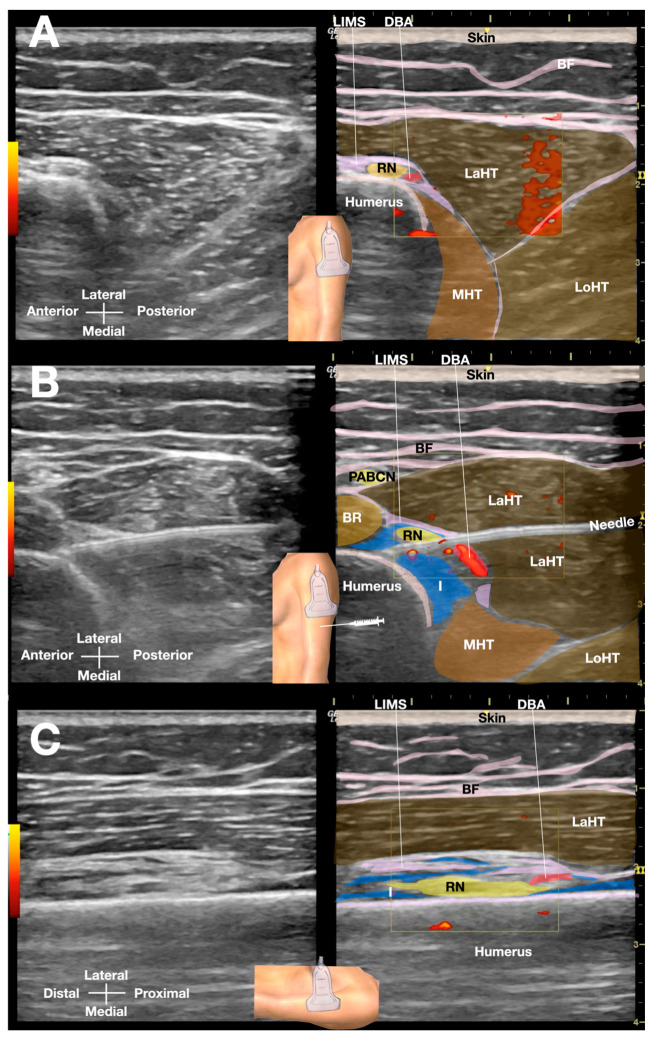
Ultrasound-guided hydrodissection of the radial nerve at the spiral groove. (A) Short-axis view showing the pre-hydrodissection sonoanatomy of the radial nerve in the spiral groove, visualizing the radial nerve adjacent to the humeral cortex and within the lateral intermuscular septum. (B) Post-hydrodissection appearance of the radial nerve, completely separated from the humerus, lateral intermuscular septum, and lateral head of the triceps by the injectate (5% dextrose in water without local anesthetics). (C) Long-axis view of the radial nerve in the spiral groove post-hydrodissection, with the injectate separating the nerve from the bony cortex of the humerus and the surrounding soft tissues. BF: brachial fascia; DBA: deep brachial artery; I: injectate; LaHT: lateral head of triceps; LIMS: lateral intermuscular septum; LoHT: long head of triceps; MHT, medial head of triceps; RN: radial nerve.
